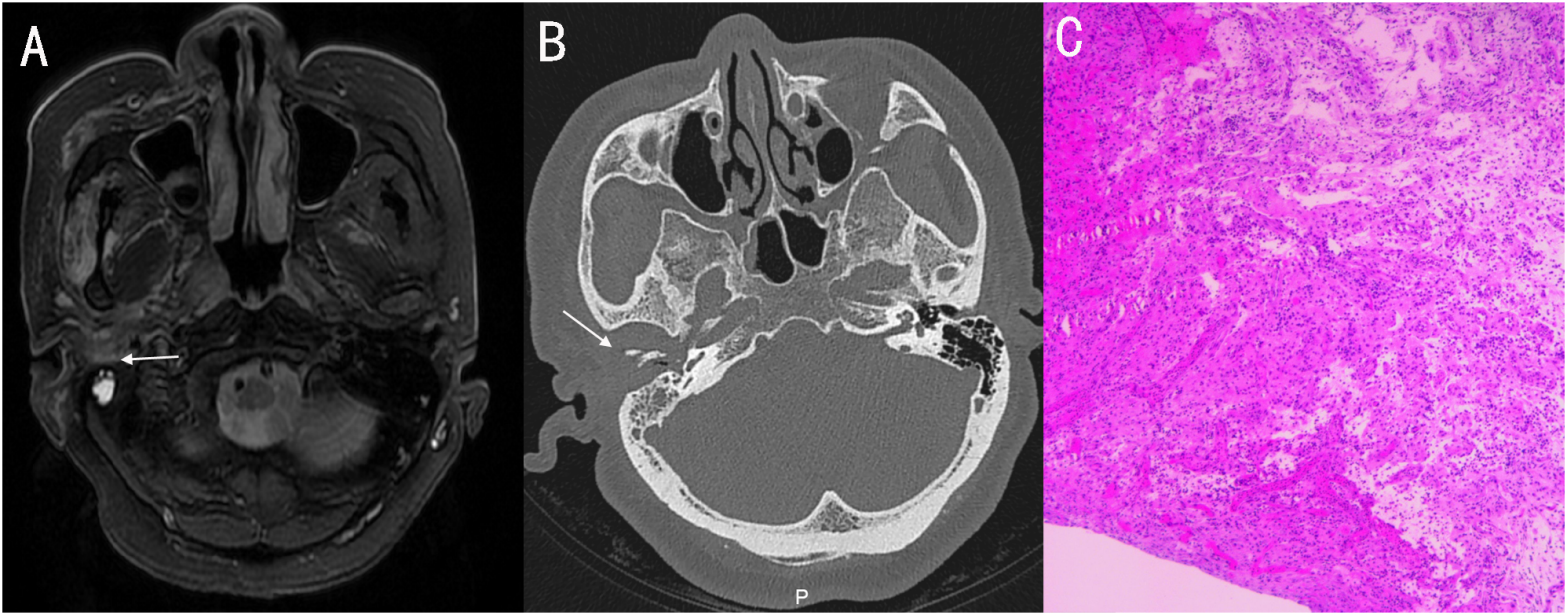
(A) MRI showed irregular tissue masses in the external auditory canal, with unclear boundaries. (B) Axial CT showing soft tissue involvement of the right external, with temporomandibular joint and eustachian tube bone erosion. (C) pathology: granulation tissue proliferation with extensive neutrophil infiltration.
